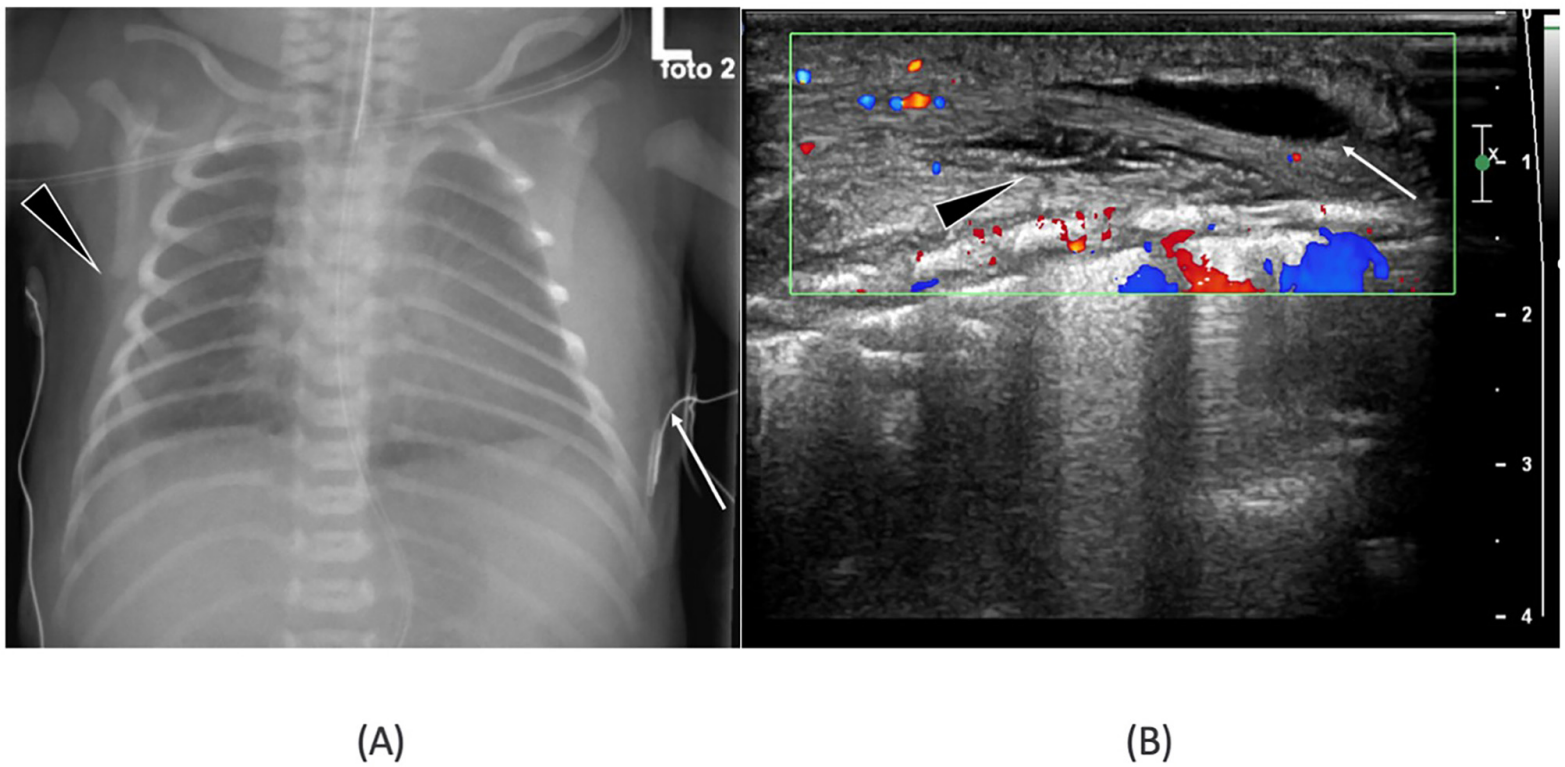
Imaging at day two. Chest wall asymmetry is observed on the left side (arrow) in the AP chest radiograph (CR) compared to the right side (arrowhead) (A). Ultrasound of soft tissue mass with high frequency probe and color doppler technique. US shows fluid collection (arrow) in the subcutaneous fat with separation of planes (arrowhead) (B).
